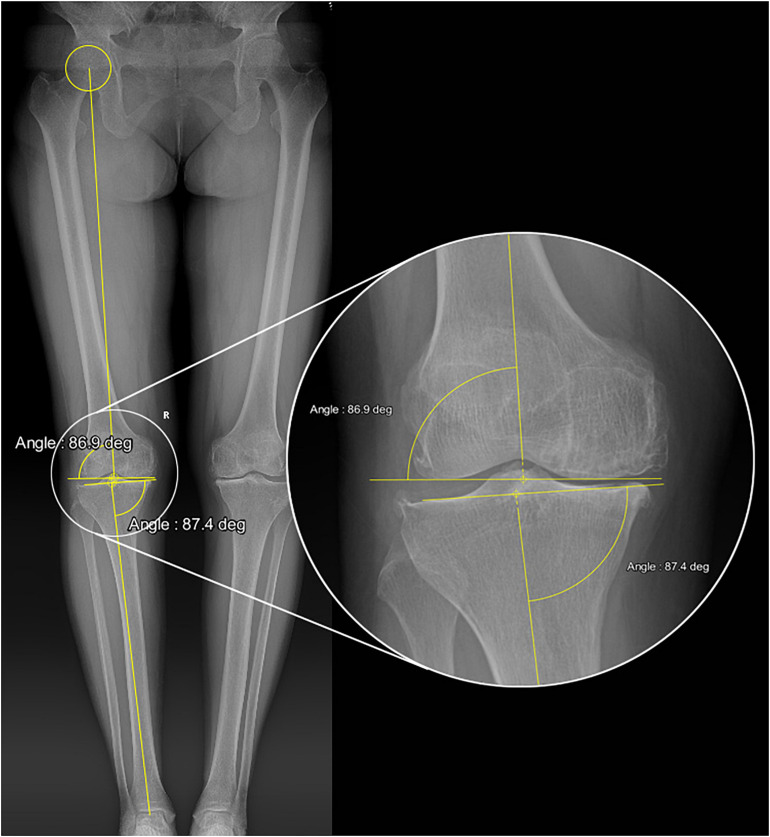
Full-length weight-bearing radiograph of the “standard” patient showing the values of mLDFA and MPTA.
